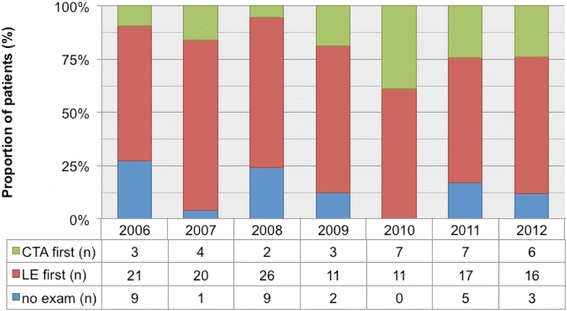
Proportion of first diagnostic intervention per year.* Computed tomographic angiography (CTA), Lower endoscopy (LE)
